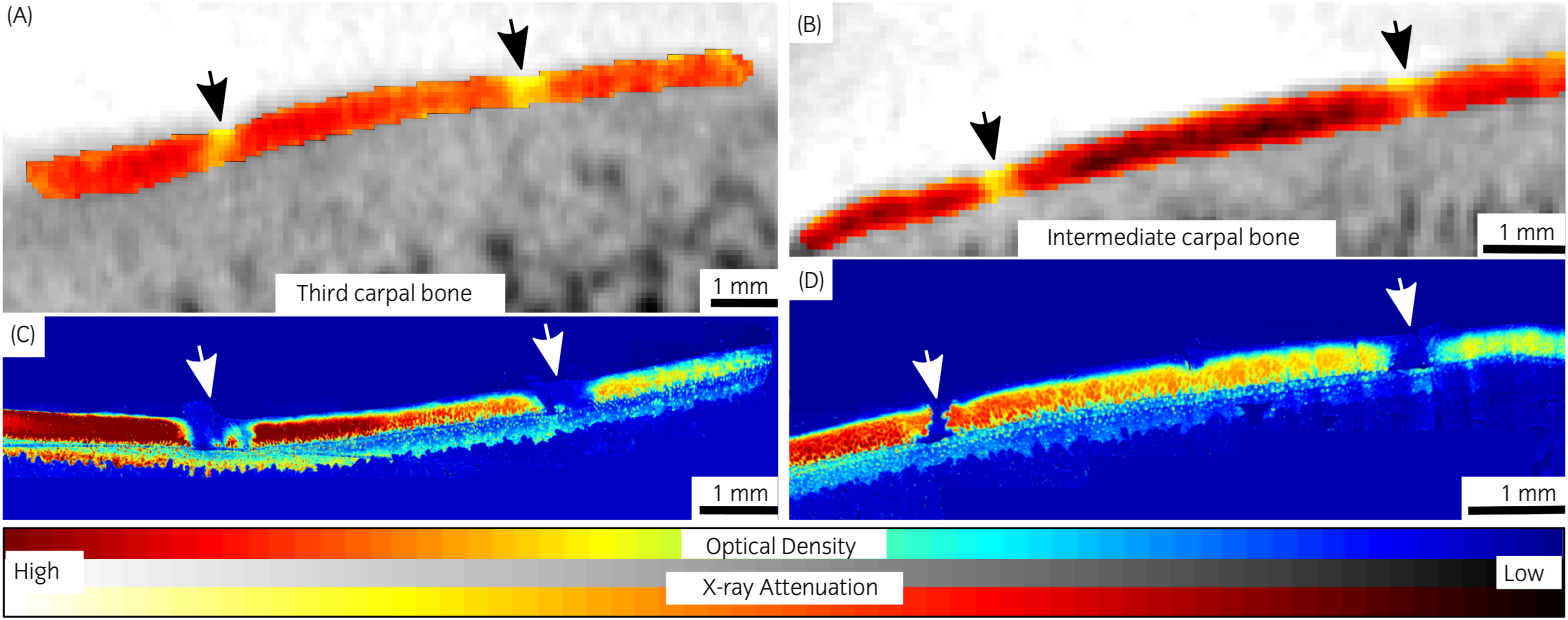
Computed tomography (CT) (A and B) and optical density (OD)‐maps (C and D) of two ponies having blunt grooves (black and white arrows in CT‐ and OD‐maps, respectively) on the articular surfaces of the third carpal bone and the intermediate carpal bone. The grooves are well detectable, and higher ioxaglate uptake near the lesion is visible in the CT images at the 60 minutes time point
